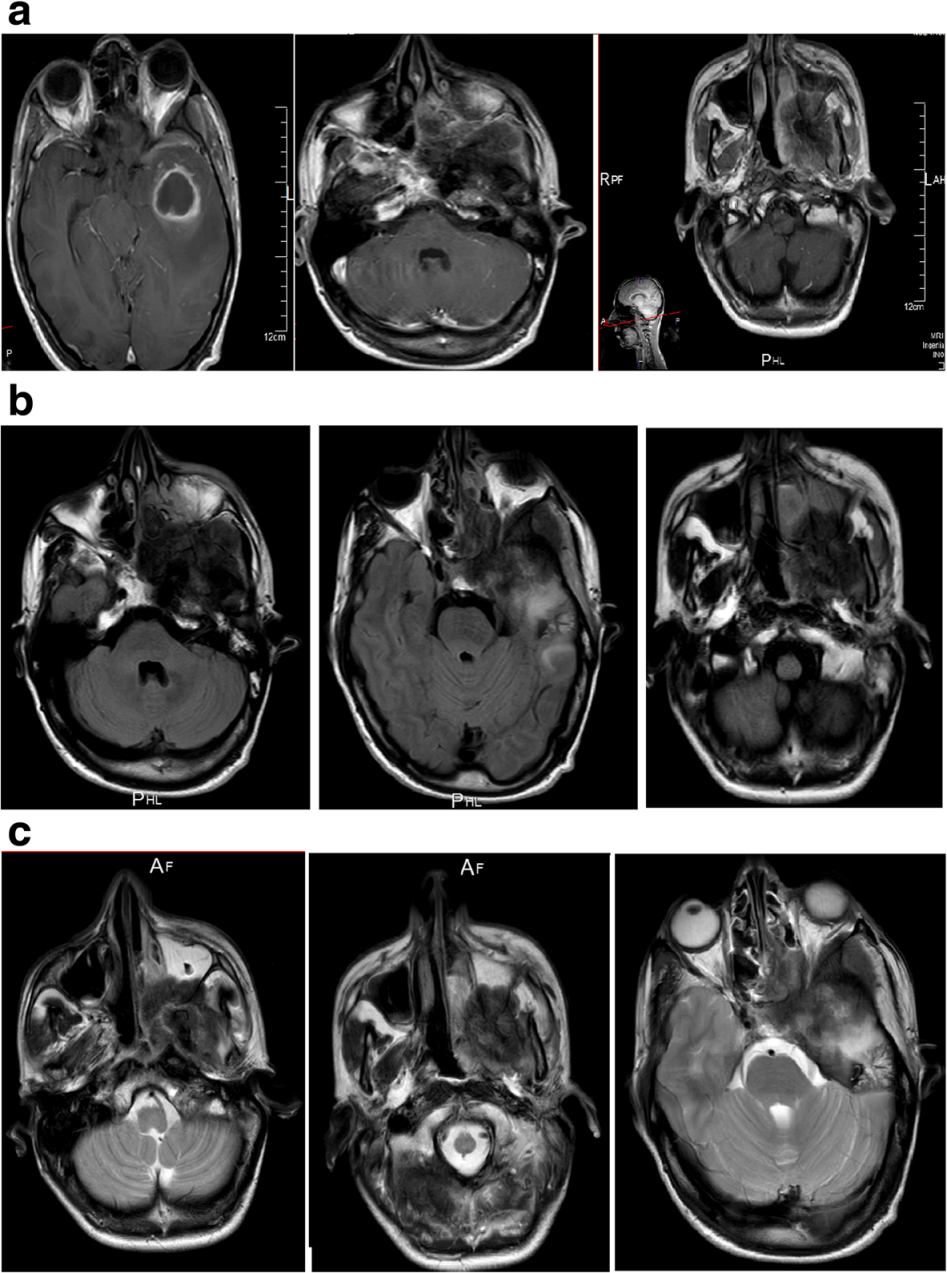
Magnetic resonance imaging studies show a tumor process of the skull base involving the sphenoid bone with its two left wings, the squamous part of the left temporal bone, associated with intracranial expansion, as well as a second, isolated mass in the temporal lobe. a T1-weighted, gadolinium-enhanced axial magnetic resonance imaging scans. b T1-weighted, axial magnetic resonance imaging scans. c T2-weighted axial magnetic resonance imaging scans
